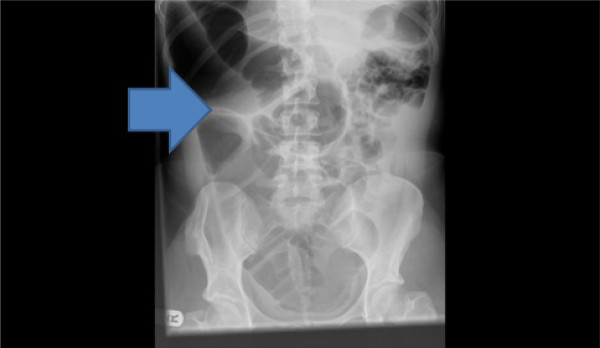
Radiograph of the abdomen showing gross dilatation of the right side of the colon.
